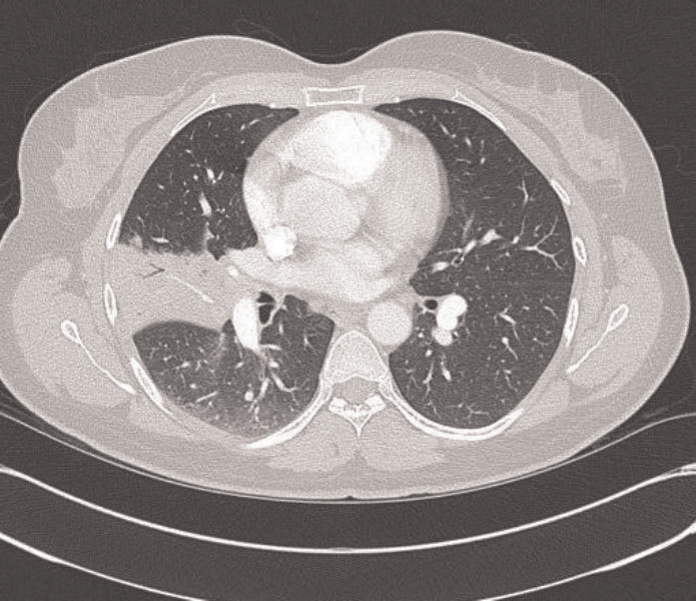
Chest CT scan demonstrating pleural thickening of the inferior half of the right middle fissure with adjacent subsegmental atelectasis of the lateral segment of the right middle lobe with some air bronchograms and bronchiectasis seen within it.
